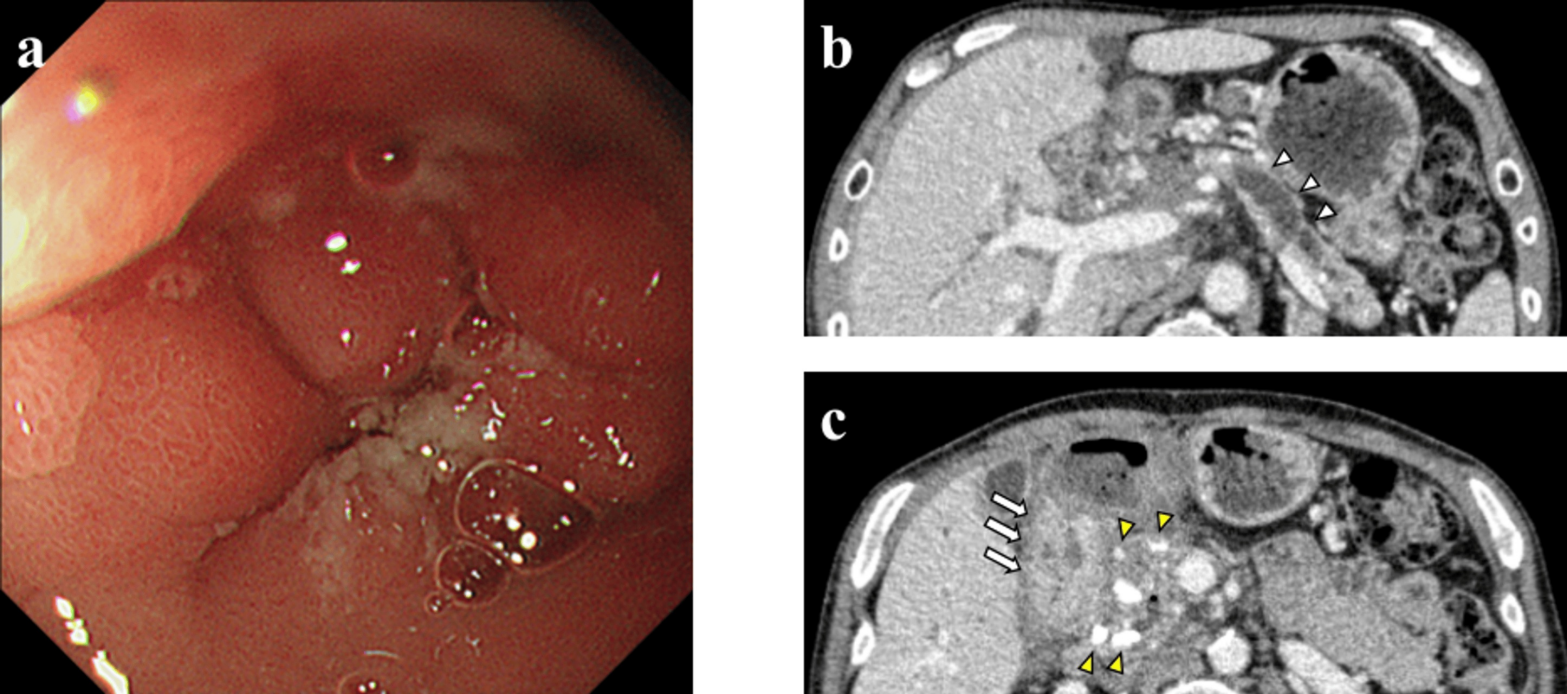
Findings of upper gastrointestinal endoscopy and preoperative CT imaging in Case 2 a: Upper gastrointestinal endoscopy showed duodenal stenosis due to ulcer scars. b: Preoperative CT imaging showed dilatation of the main pancreatic duct (white arrowheads). c: Pancreatic stones (yellow arrowheads) and thickening of the duodenal wall were observed (white arrows).
